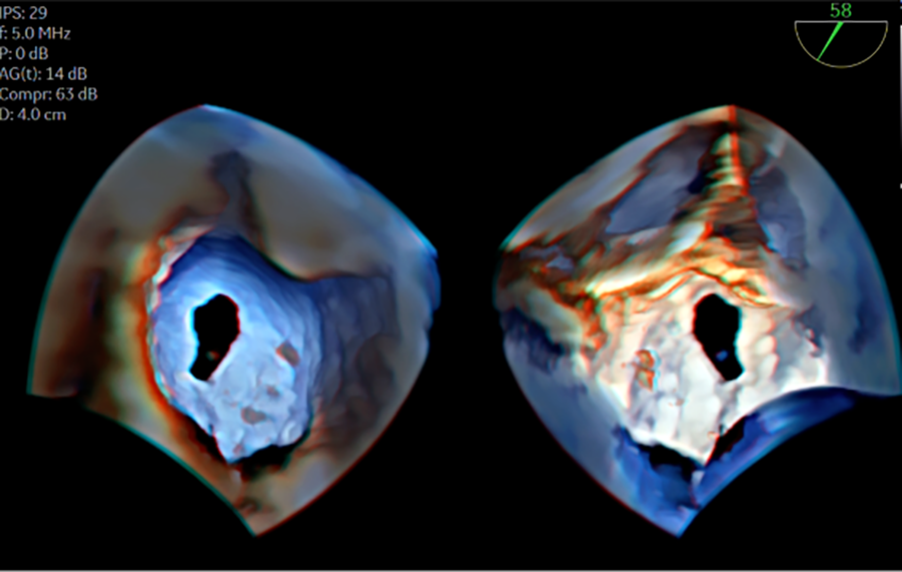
3D TOE in a 18 kg patient with ostium secundum ASD. Transillumination rendering in dual cropping views with FlexilightTM showing en face view of the ASD from the RA (left panel) and the LA (Supplementary Video S10). 3D, three-dimensional; ASD, atrial septal defect; LA, left atrium; RA, right atrium; TOE, transoesophageal echocardiography.
